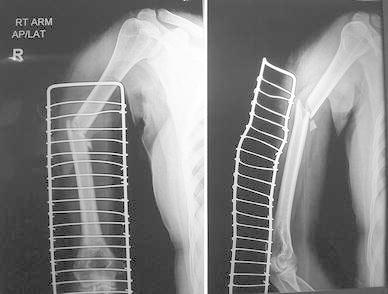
AP and lateral radiographs of fracture middle third of the shaft of humerus
